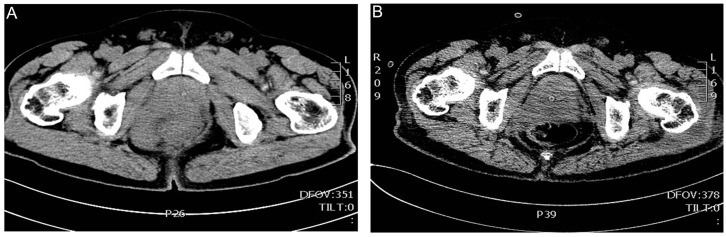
Computed tomography (CT) scans prior to and following treatment. (A) CT scan clearly showing a prostatic space-occupying lesion with a maximum intersecting surface 8.3×7.1 cm in size, fuzzy boundaries with the rectum and an absence of the bladder seminal vesicle angle prior to treatment. (B) Following treatment, the prostate was almost back to normal, with relatively clear boundaries with the rectum.
